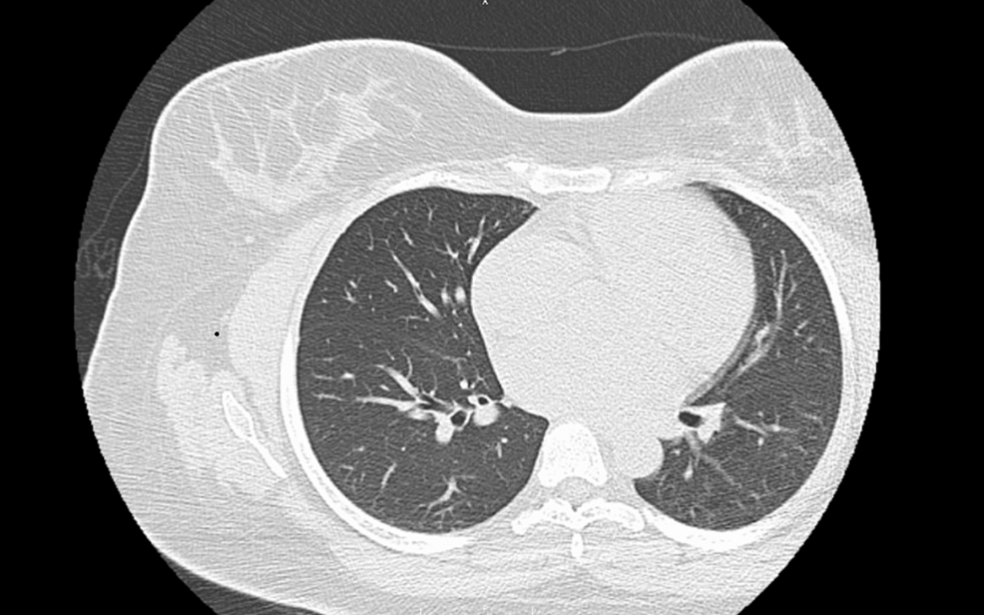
Computed tomography of the chest without contrast portrays complete resolution of the bilateral ground-glass opacities
